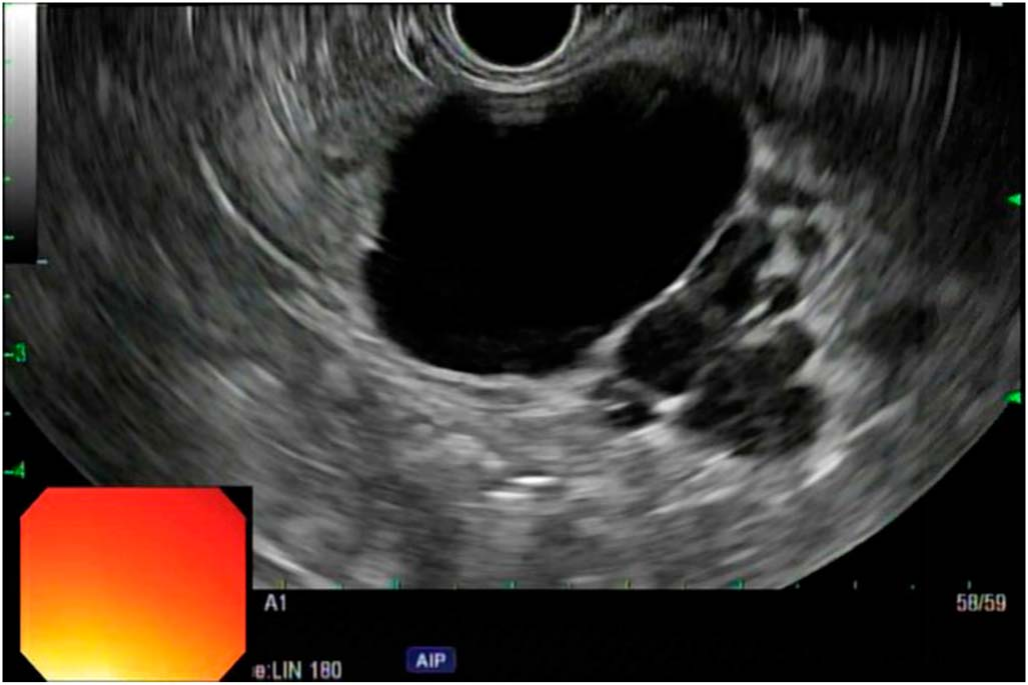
Endoscopic ultrasound (EUS) imaging of the cystic transformation of the native pancreas.
